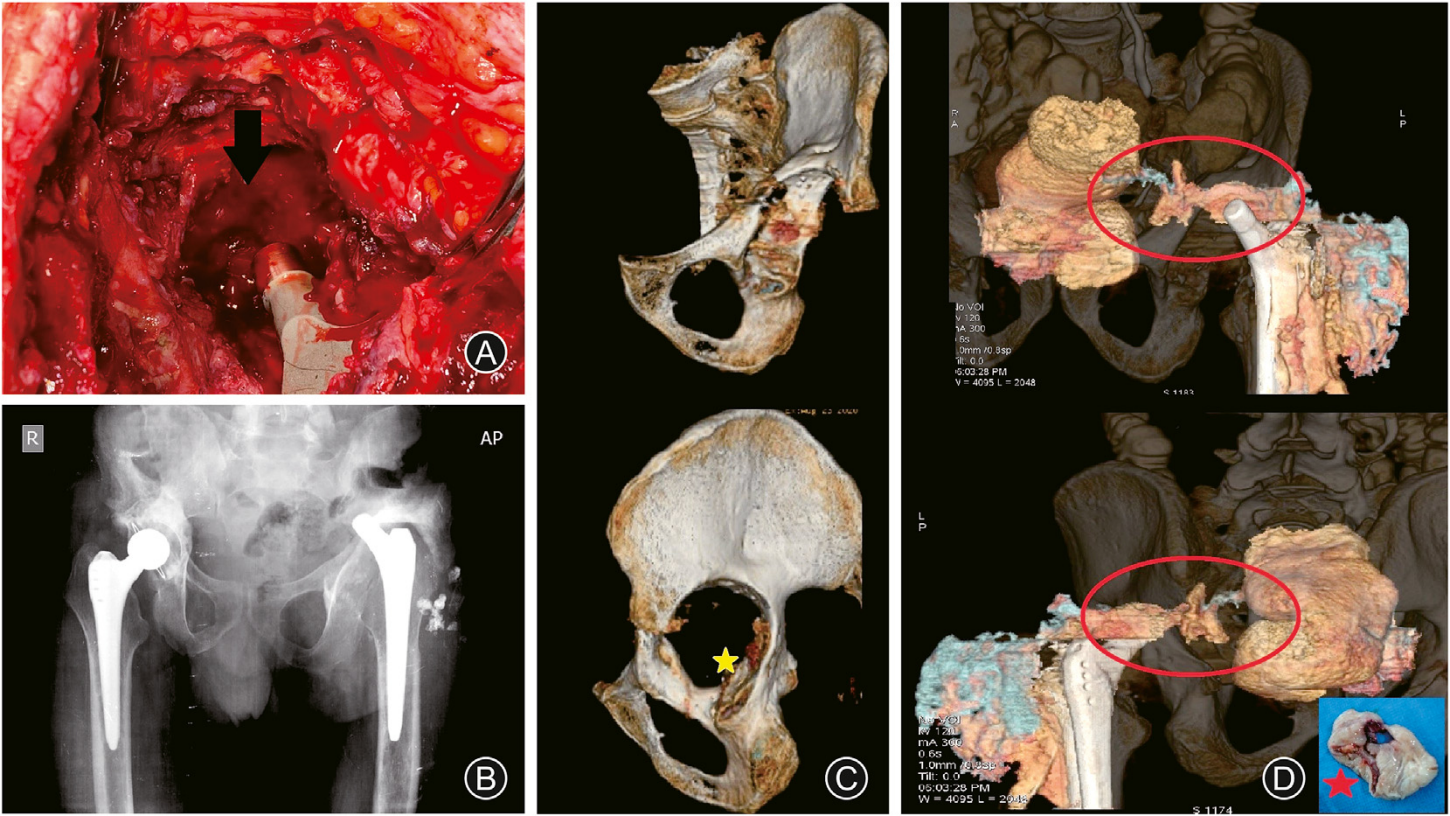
Detecting the presence of coloarticular fistula. (A) and (B) Intraoperative and postoperative images (radiographs and CT) showing acetabular floor deficiency type ⅡC (yellow asterix) and retained stem; (D) CT scan with contrast showing the coloarticular fistula (red circle) and after fistulas tract excision (red asterix).
